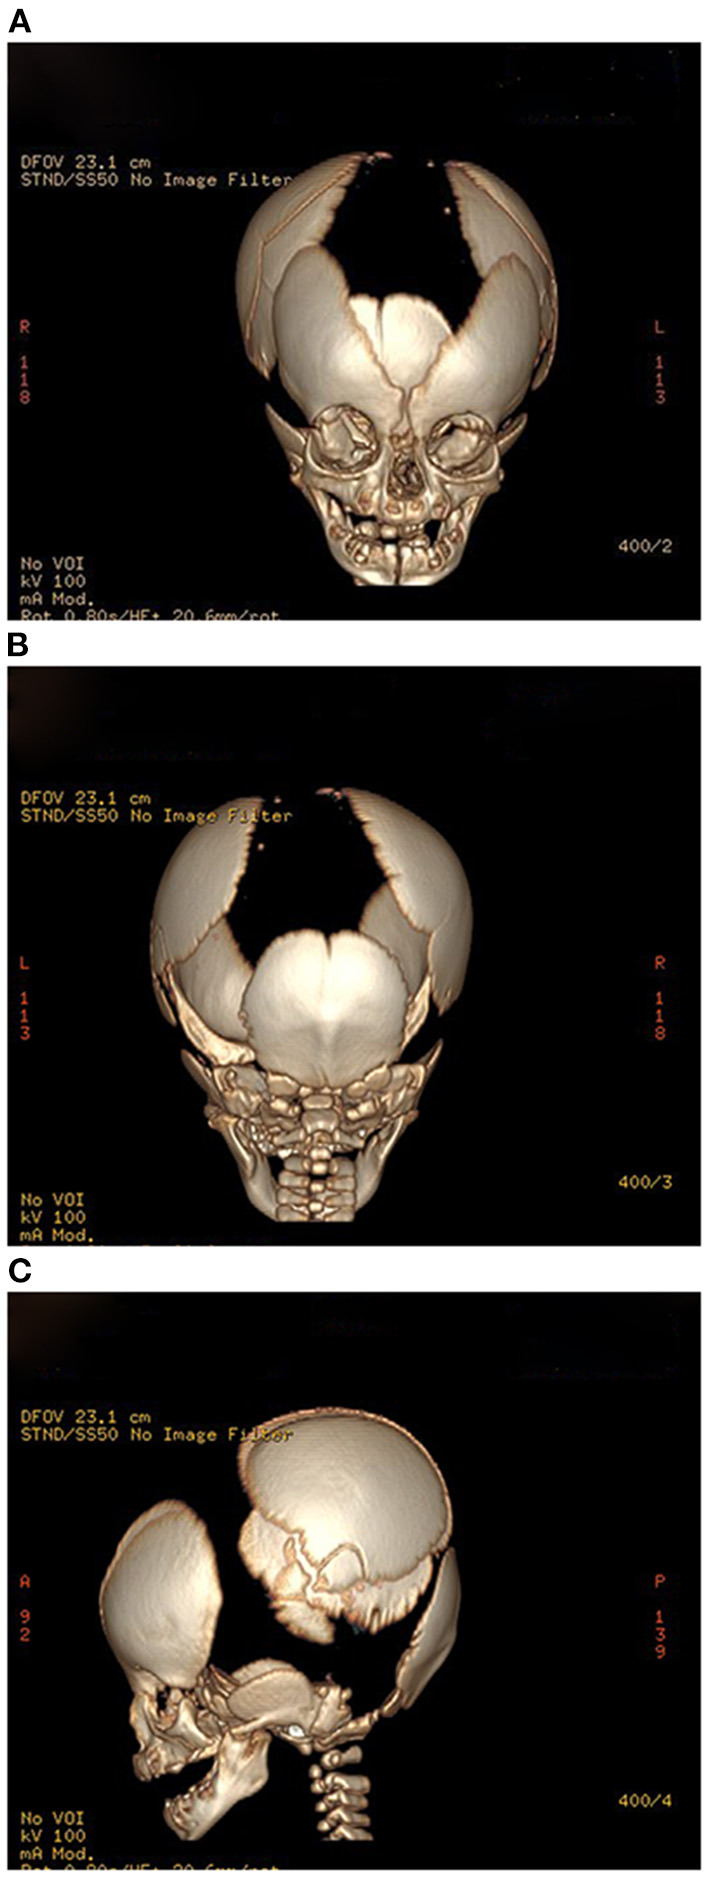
(A–C) Propositus skull CT, frontal, posterior, and lateral views: delayed ossification and wide-open sutures.
